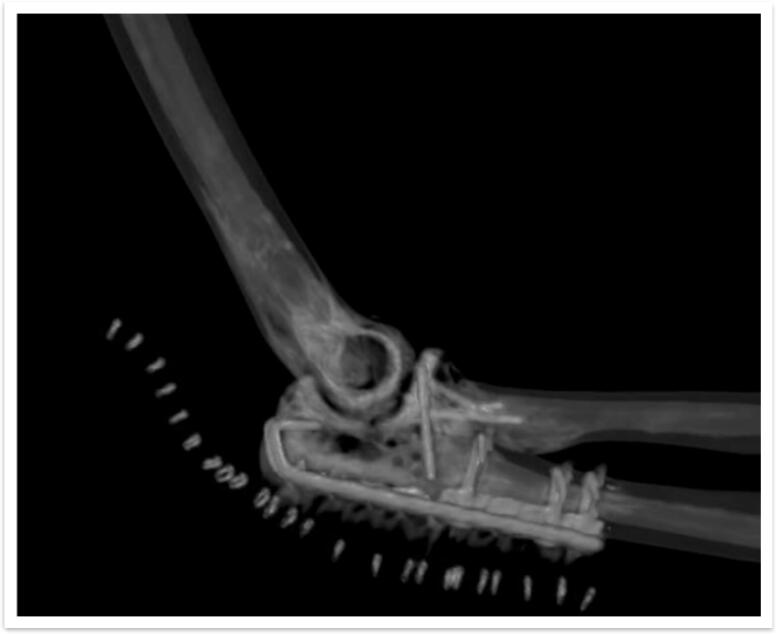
3D reconstruction of postoperative CT scan, with osteosynthesis material in red, showing adequate reduction of the articular surface of the elbow with all material in subchondral bone without affecting the biomechanics of the joint. (For interpretation of the references to colour in this figure legend, the reader is referred to the web version of this article.)
